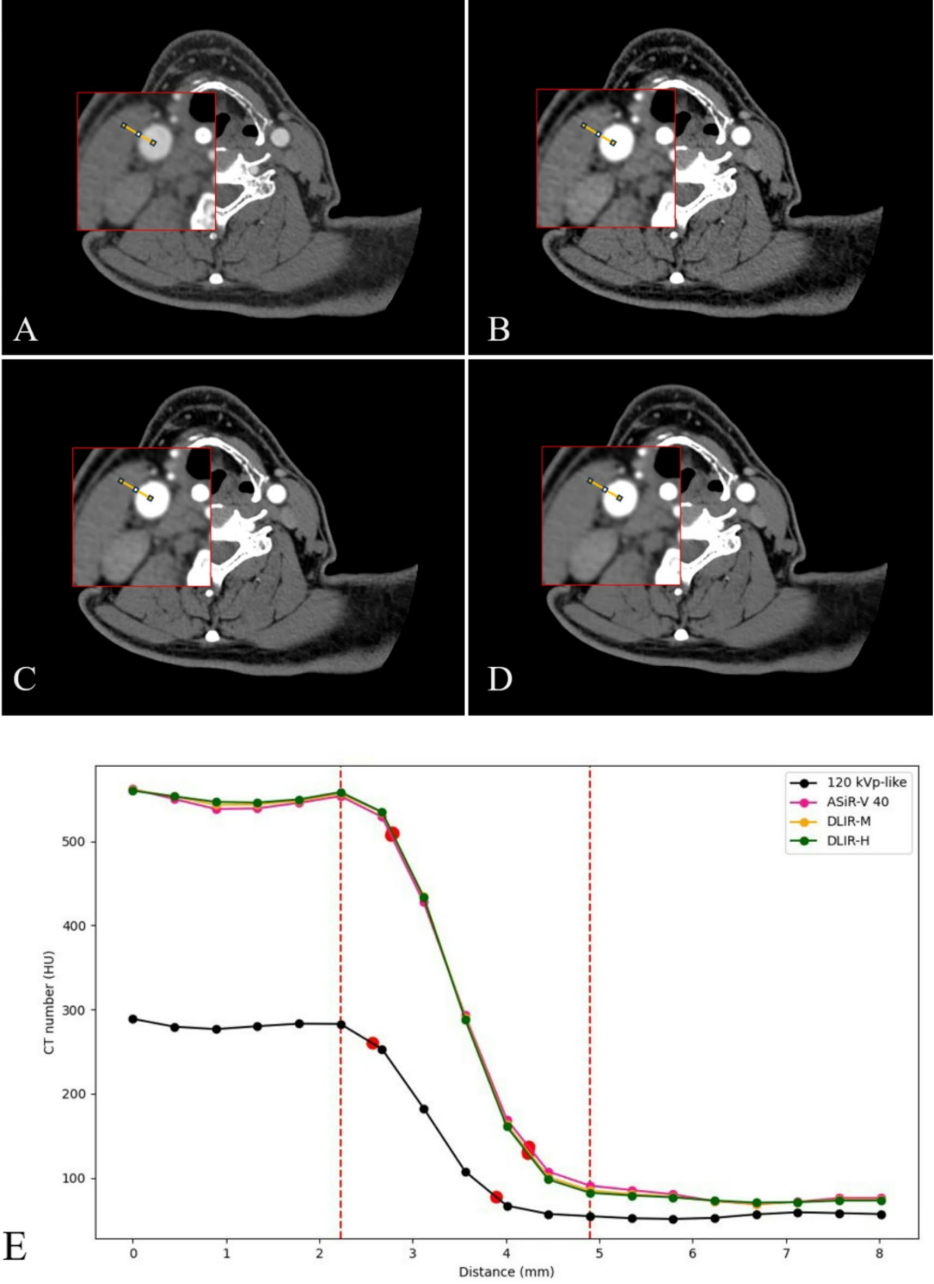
Examples of quantitative measurement used in our study. (A) reconstructed images from 120-kVp-like with ASIR-V40%; (B) reconstructed images from 50 keV with ASIR-V40%; (C) reconstructed images from 50 keV with DLIR-M; (D) reconstructed images from 50 keV with DLIR-H; E, ERD and ERS of four reconstruction algorithms. The common carotid artery running in the axial plane was selected, and a perpendicular line was drawn (the yellow lines in A - D), edge line profile was calculated reflecting the CT attenuation profile of those lines. In figure E, the red dashed lines in figure E indicated the last dip point and the first peak point on the rapid descending CT attenuation curve, and the red dots indicated the 10% point and 90% point. ERD was the distance between two red dots in its CT attenuation curve and ERS was the slope between these two red dots
